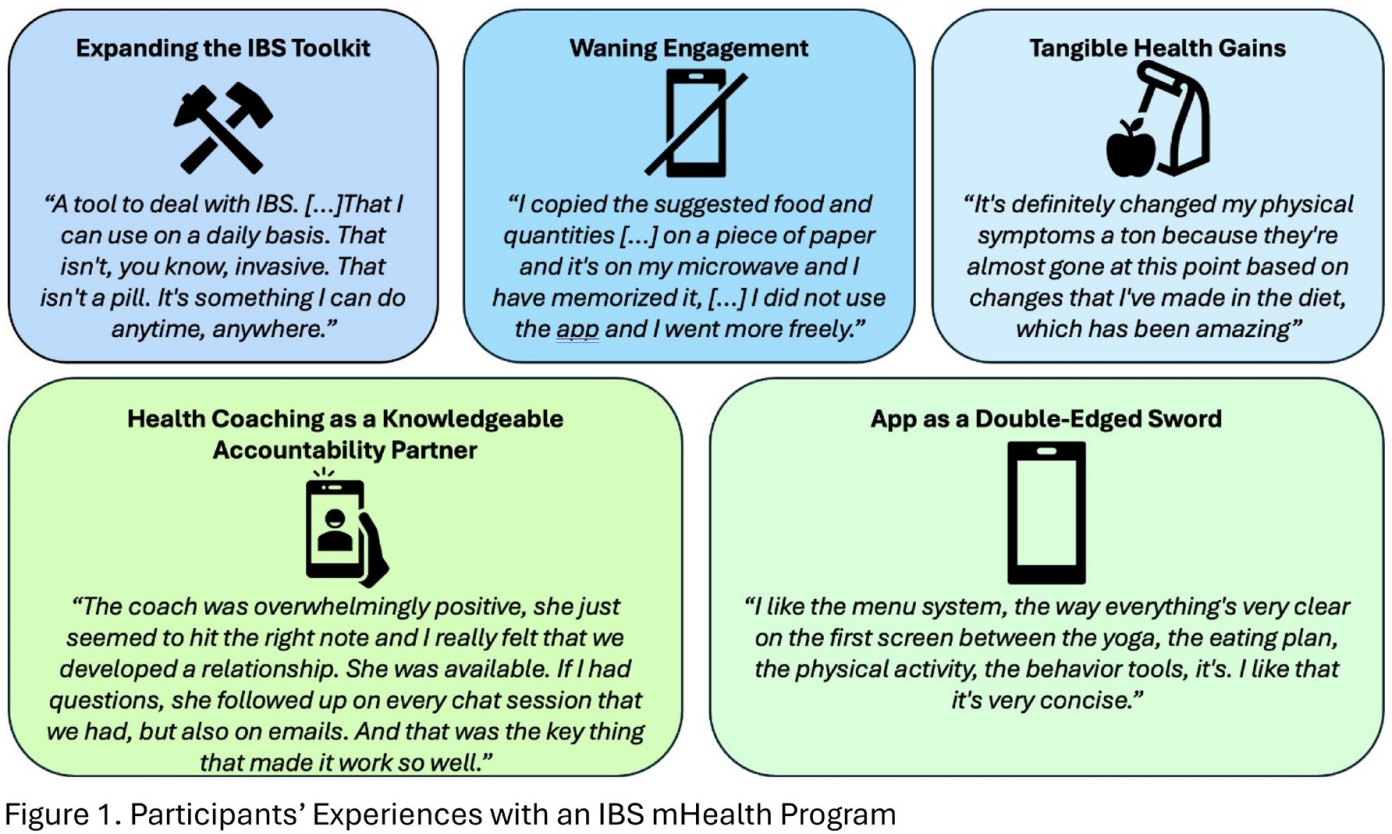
# Poster Session I - A149 EMPOWEREMENT IN THE PALM OF YOUR HAND: IBS PATIENT PERSPECTIVES ON A MOBILE APP WITH HEALTH COACHING
